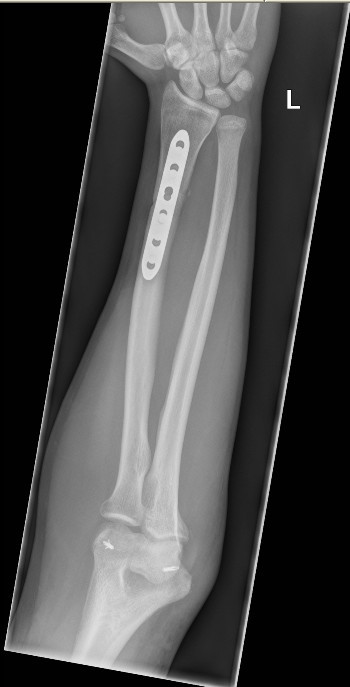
A postoperative X-ray gives an anteroposterior (AP) view after open repair of collateral ligaments.
